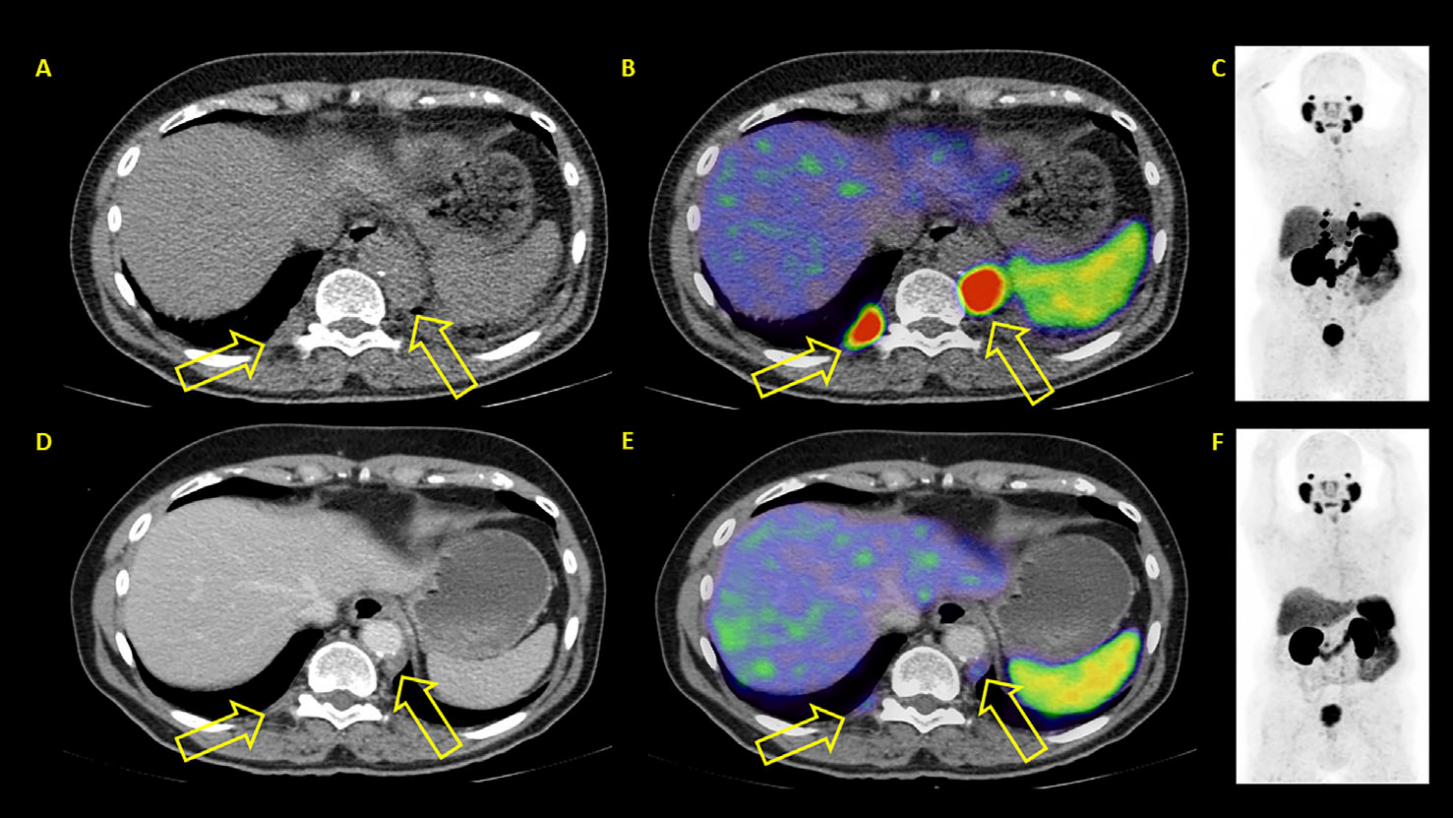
Example of a patient where both PSA and PET showed similar results: partial remission. After two therapy cycles PSA values decreased from 46.4 to 1.42 ng/ml. PET/CT also showed partial remission. (A–C) represent baseline images whereas (D–F) represent interim PET/CT. (A, D): axial CT slices; (B, E): axial fused PET/CT images; (C, F): maximum intensity projection images.
